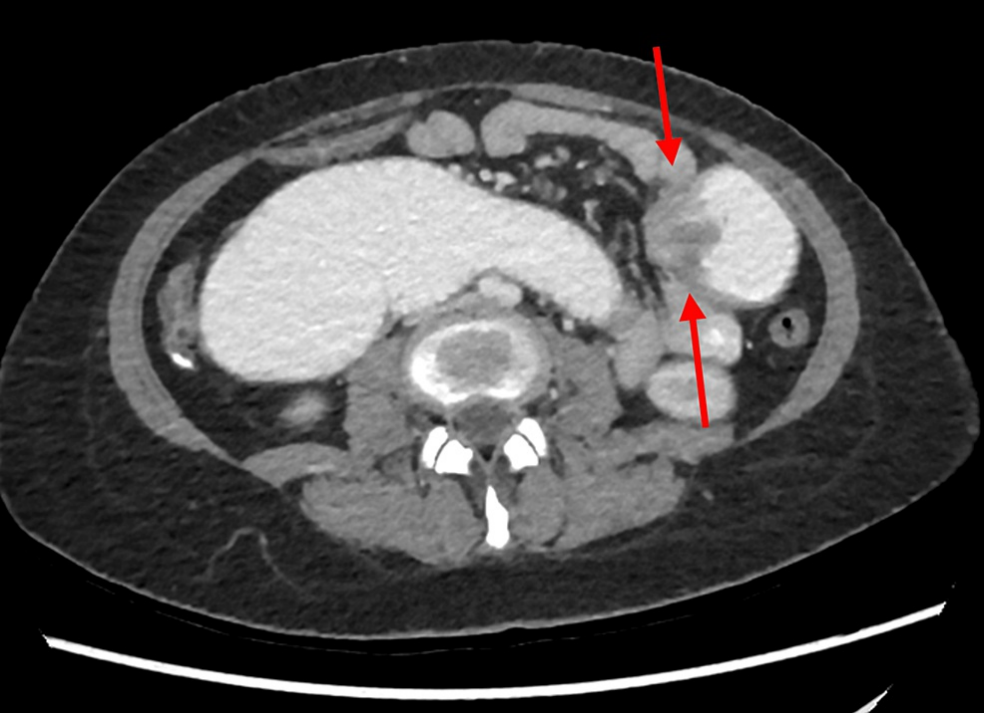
An abdomen computed tomography scan with contrast showing a short segment of irregular circumferential mass involving the proximal part of the jejunum.
